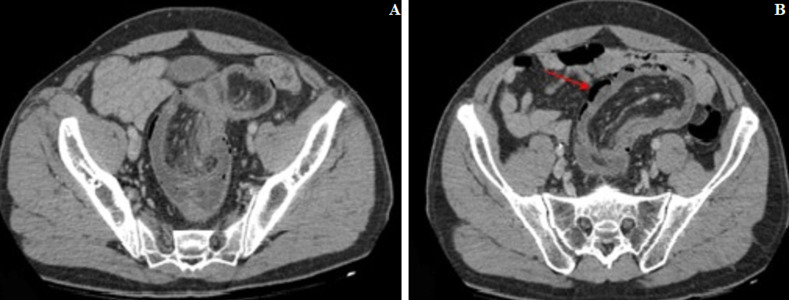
axial view of a CT scan of the abdomen showing the colo-rectal intussusception (A) with invaginated mesenteric fat and parietal pneumatosis (redarrow) (B)
